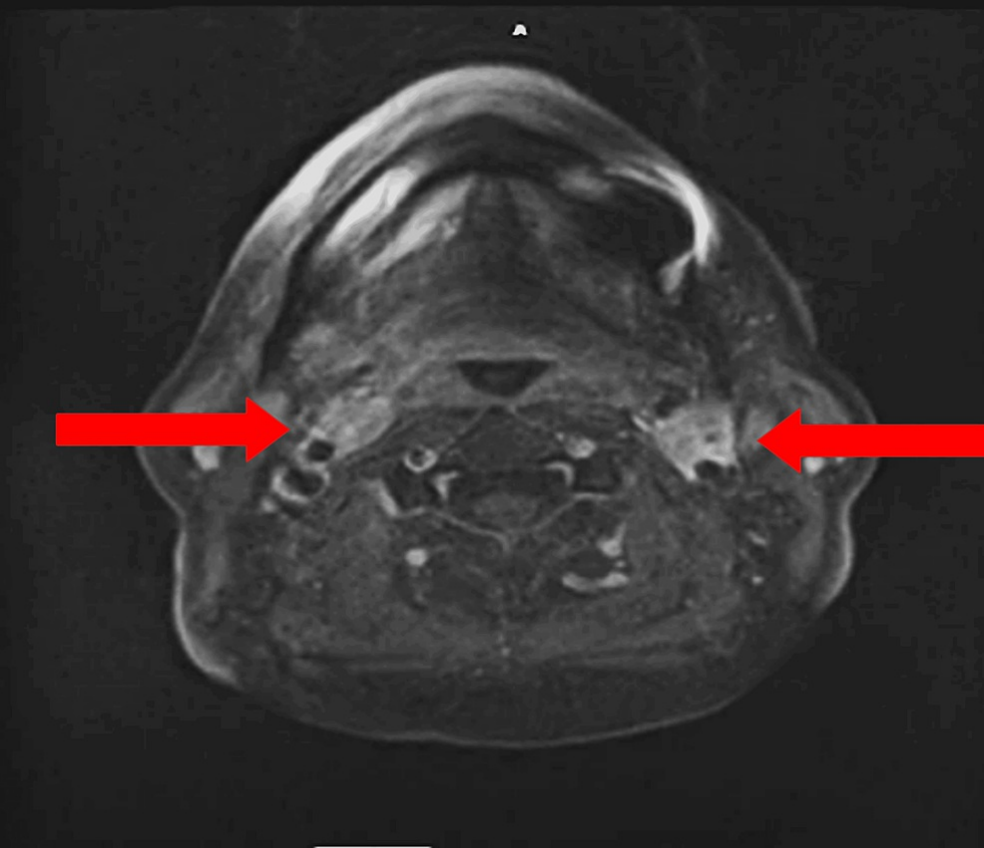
Axial T1 contrast-enhanced MRI cross-section showing enhancing bilateral carotid body tumors with splaying of the internal and external carotid arteries (red arrows)
